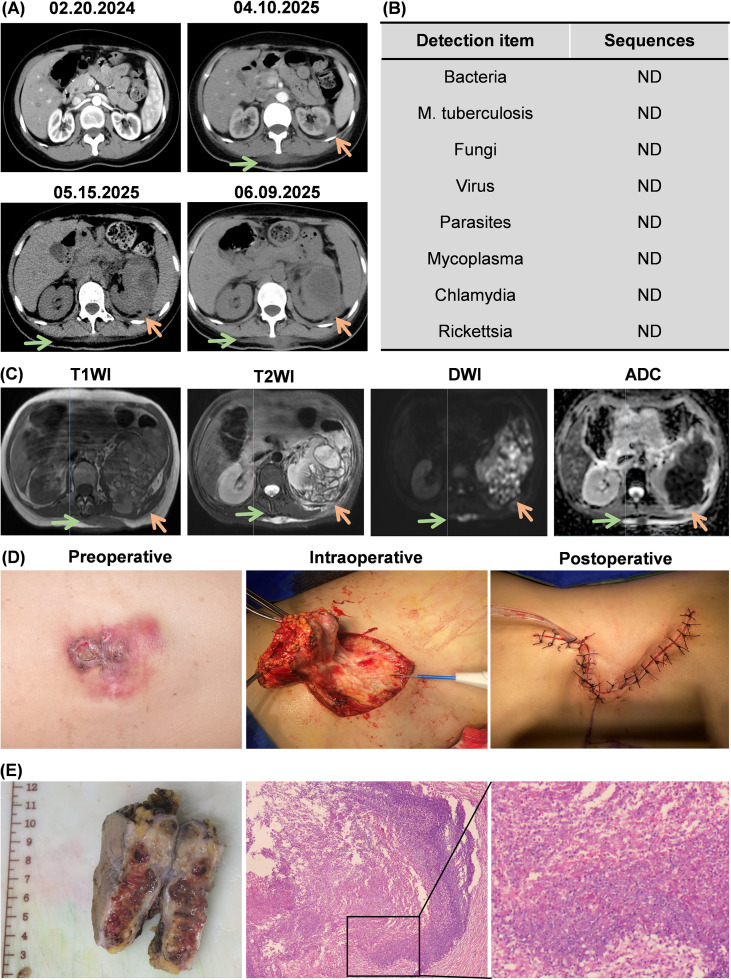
Examination and surgical management of aseptic inflammatory abscesses of the back and nephritic abscess. (A) abdominal continuous Computed Tomography (CT) Scan: February 20, 2024—no abnormalities in the back and the left kidney; April 10, 2024—initial detection of inflammatory abscesses of the back and nephritic abscess; May 15, 2024 - June 9, 2025: Progressive enlargement of the inflammatory abscesses in the back and nephritic abscess; (B) High-throughput microbial DNA testing of back puncture fluid: Negative results, indicating a aseptic inflammatory abscesses of the back; (C) July 2025 Magnetic Resonance Imaging (MRI) examination: The aseptic inflammatory abscesses of the back and nephritic abscess showed low signal intensity on T2-weighted images (T2W1) and high signal intensity on T1-weighted images (T1W1) within the cyst wall, along with diffusion-weighted imaging (DWI) showing diffusion suppression and corresponding apparent diffusion coefficient (ADC) mapping; (D) Preoperative, intraoperative, and postoperative findings of the aseptic inflammatory abscesses of the back. Intraoperative examination revealed a multilocular cystic abscess-like lesion. Complete surgical resection of the aseptic inflammatory abscess of the back region was achieved; (E) Pathological image of the aseptic inflammatory abscesses of the back: Chronic inflammatory changes were observed, with no malignant tumor cells present. ND, Not Detected; T1WI, T1-weighted imaging; T2WI, T2-weighted imaging; DWI, diffusion-weighted imaging; ADC, apparent diffusion coefficient.
